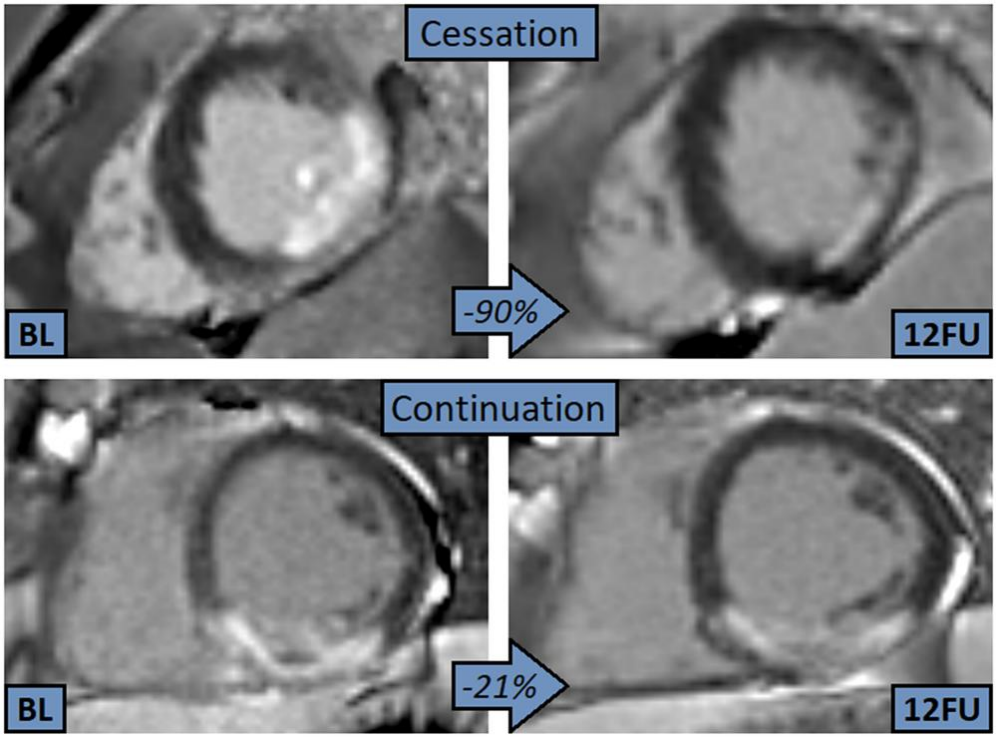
Infarct size evolution over 12 months (left LGE image: baseline CMR, right LGE image: 12 month follow-up) in two patients with markedly different infarct size reduction within the first year after myocardial infarction: the patient in the top row (inferolateral infarction) quitted smoking after the index event, whilst the patient in the lower row (inferoseptal infarction) continued smoking. Percentages represent the relative decrease in infarct size between examinations. *12FU: 12-month follow-up, BL: baseline, CMR: cardiac magnetic resonance imaging.*
